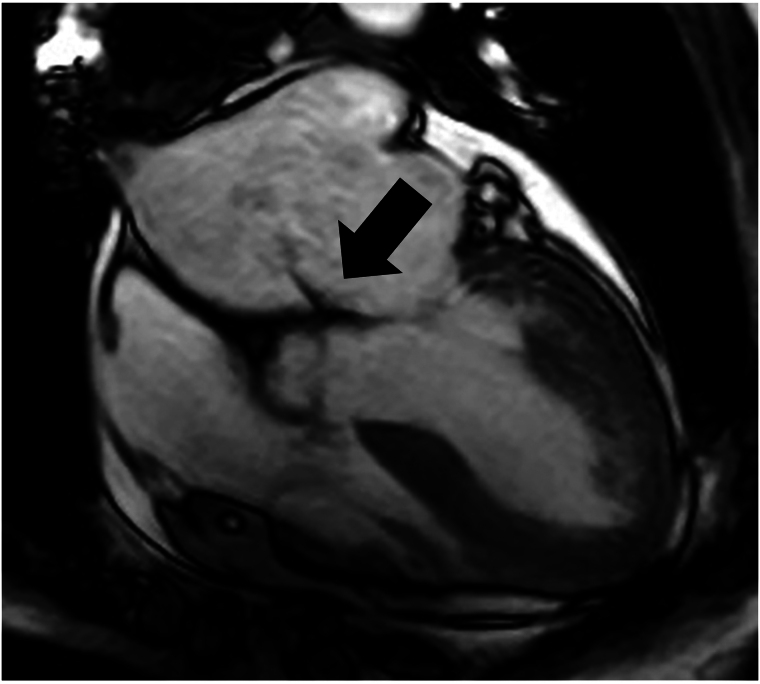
Cardiac magnetic resonance imaging (CMR). CMR cine showed a fast narrow jet through anterior mitral leaflet (arrow).
